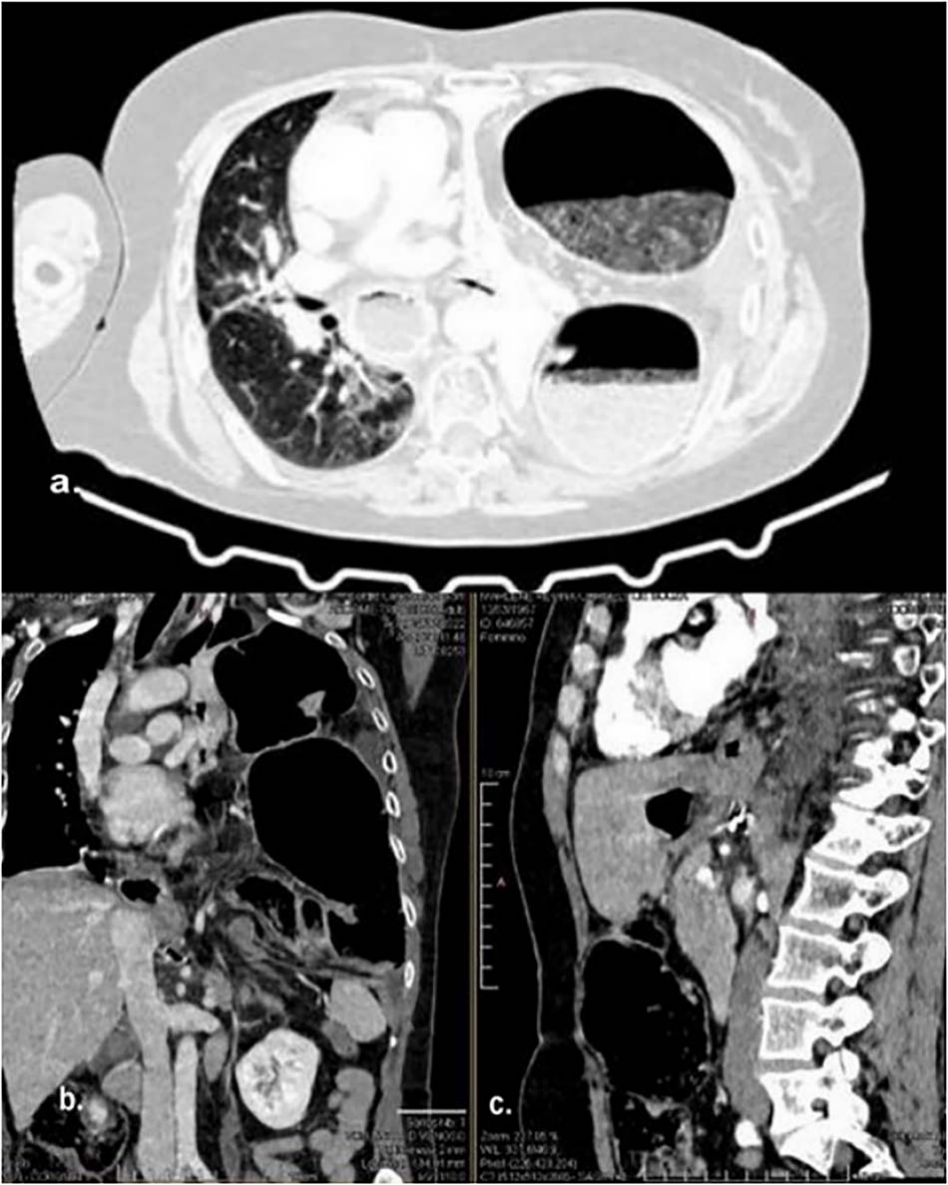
Different sections of the CT study demonstrate the mediastinal position of the transversal volvulus. (a) Axial CT section of the abdomen showing signs of esophagectomy with gastric lifting, a type IV hiatal hernia and colon herniation into the thorax. (b) The coronal CT section of colon herniation into the thorax. (c) The sagittal CT section of the thorax and the abdomen shows the posterior mediastinal position of the colon herniation into the thorax.
